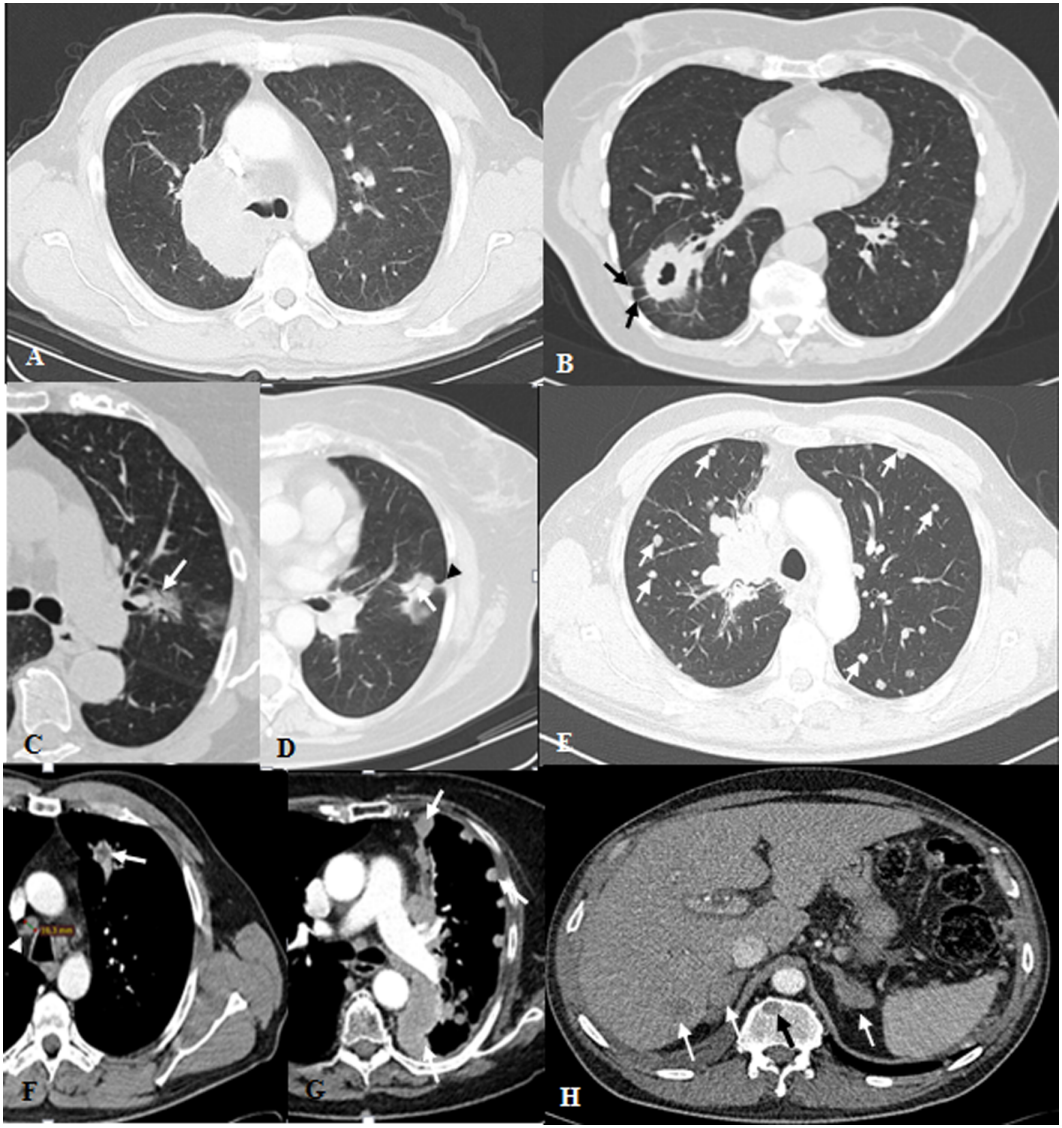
Transversal CT images of pulmonary adenocarcinoma with examples of the morphological features under investigation. (A) Central lesion with tumor-bearing lobe atelectasis; (B) peripheral cavitary solid mass with ovoid shape, predominant spiculated margins and associated pleural tags (arrow); (C) central irregular semi-solid lesion in smooth margin with air bronchogram (arrow), GGO account for 25%-50%; (D) peripheral lesion with irregular shape, air bronchogram (arrow) and pleural tag (arrowhead); (E) central solid lesion with irregular shape and predominant lobulated margins with bilateral pulmonary metastases (arrows); (F) Irregular shape tumor after contrast enhancement showed central necrosis (arrow) and contralateral mediastinal lymph node metastasis (arrowhead); (G) tumor-side pleural metastases (arrows); (H) liver, bone, and bilateral adrenal gland metastases (arrows).
